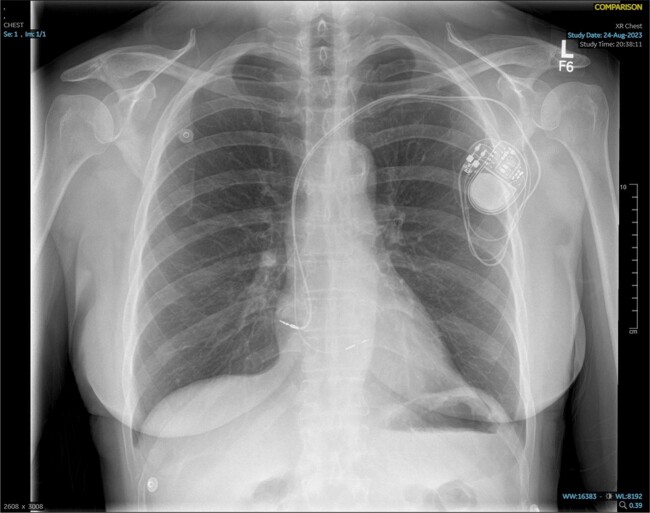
Chest X-ray (performed 2 h after left bundle branch area pacing) shows a dual-chamber permanent pacemaker with left bundle branch area pacing. A 69 cm SelectSecure lumenless 3830 lead was placed in the deep interventricular septum while a 52 cm CapSureFix Novus 4076 lead was positioned in the right atrial appendage.
